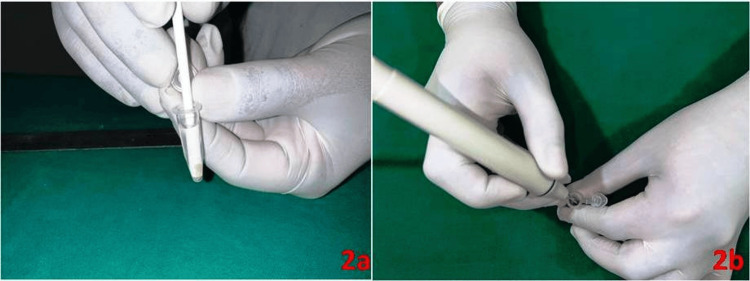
Activation methods used in the study (2a) Manual digital agitation (MDA) and (2b) ultrasonic (US) method.
